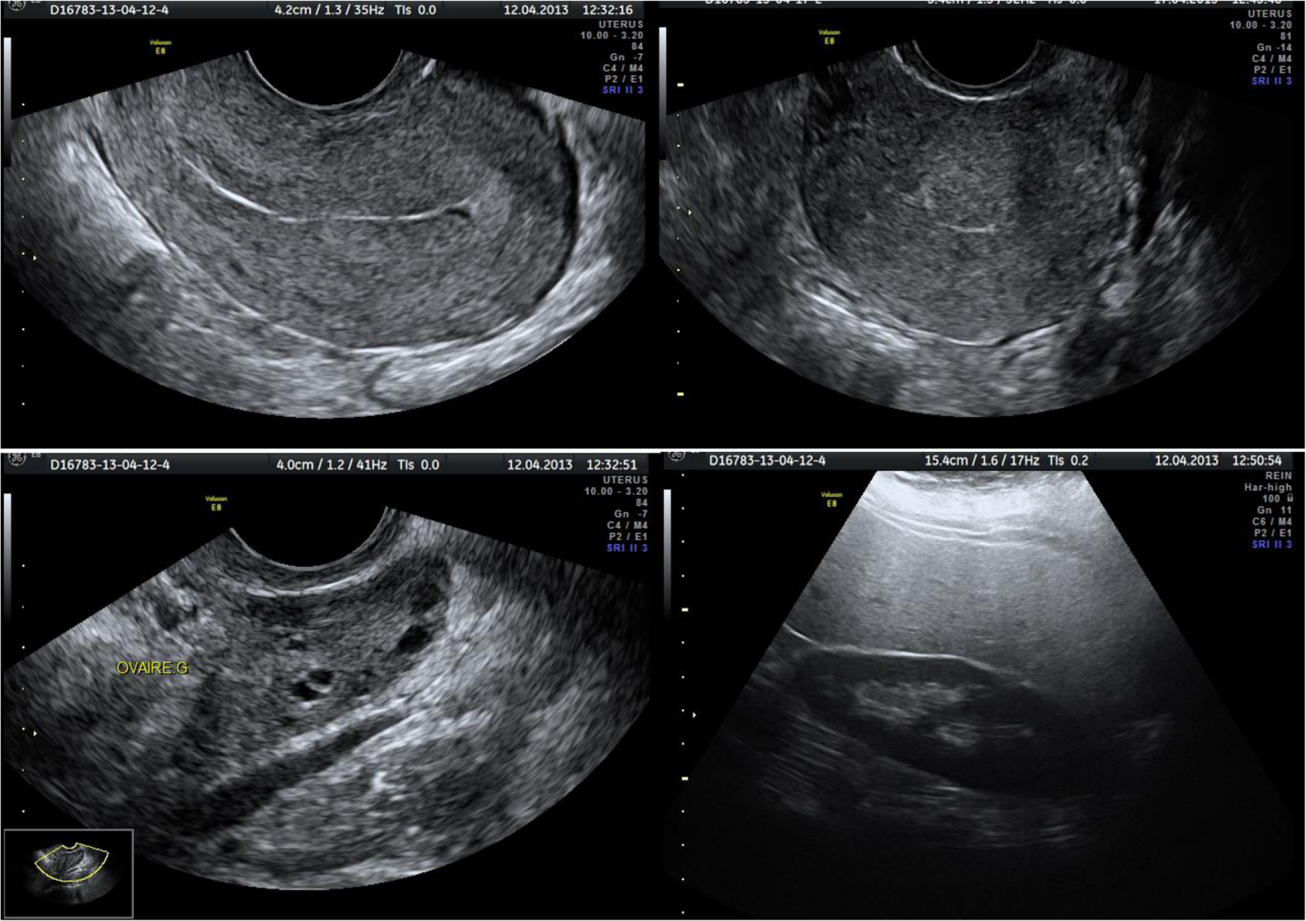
Standardized ultrasonography scans. (i) longitudinal view of the uterus, (ii) transverse view of the uterus, (iii) view of left ovary, and (iv) view of Morison’s pouch.
